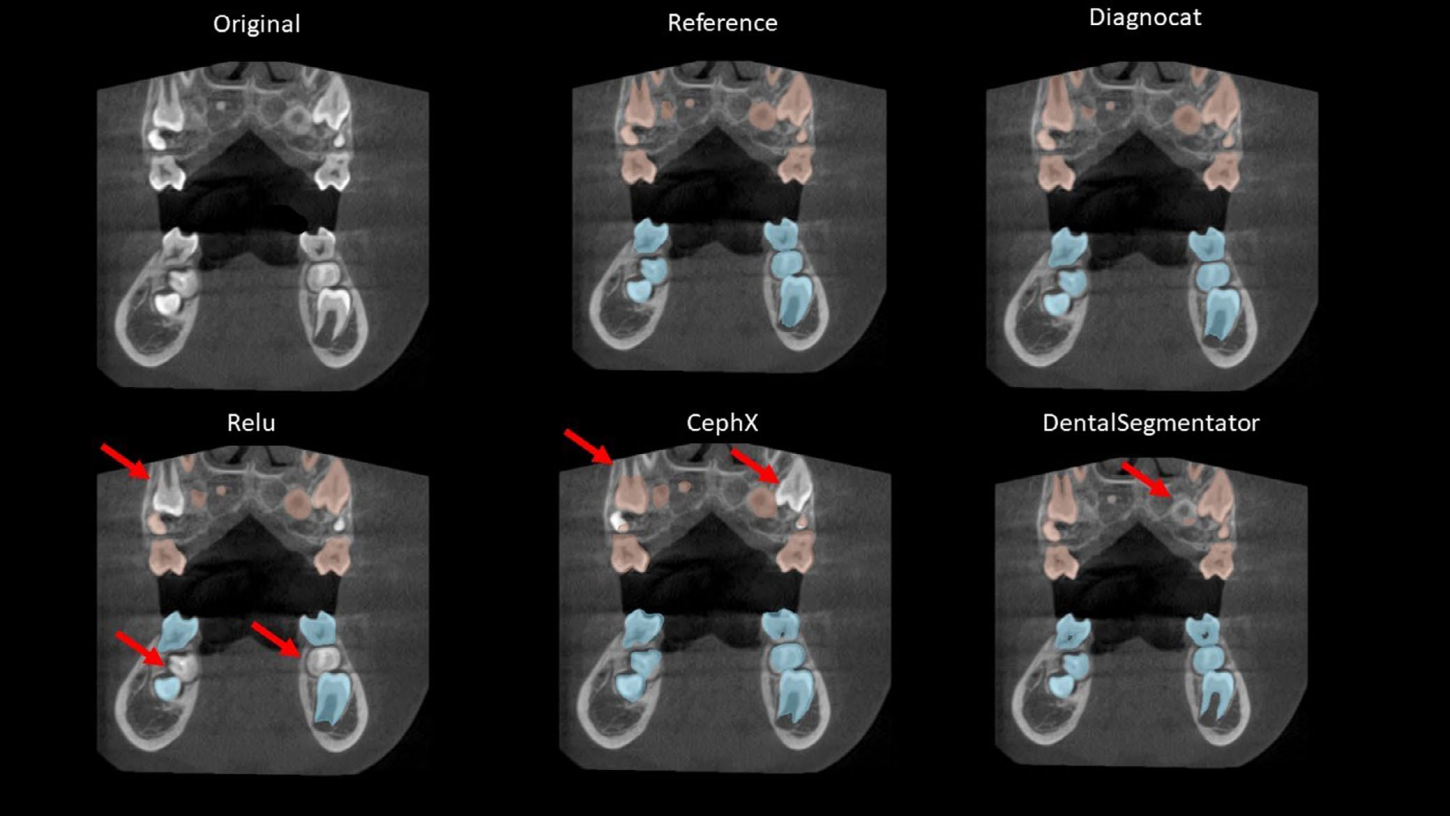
A representative case (Case C from Figure 2) showing a frontal section of the original CBCT, the reference segmentation and the DL segmentations. The maxillary teeth are colored in red, and the mandibular teeth are in blue. Red arrows signify under‐segmented regions.
